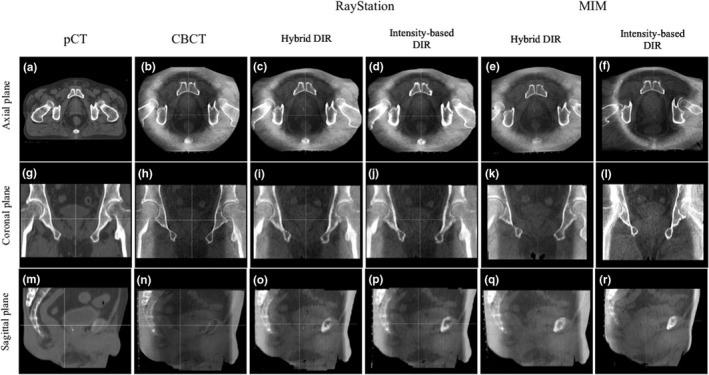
Comparison of the deformed CT images between the hybrid and intensity‐based deformable image registration (DIR) in Patient 1. The left two columns show the planning CT and the cone‐beam computed tomography images as a reference and a target for the DIR. The other four columns show images deformed by the hybrid and intensity‐based DIR algorithms. Patient 1 was a good case to understand the usefulness of the hybrid DIR in both commercial algorithms.
